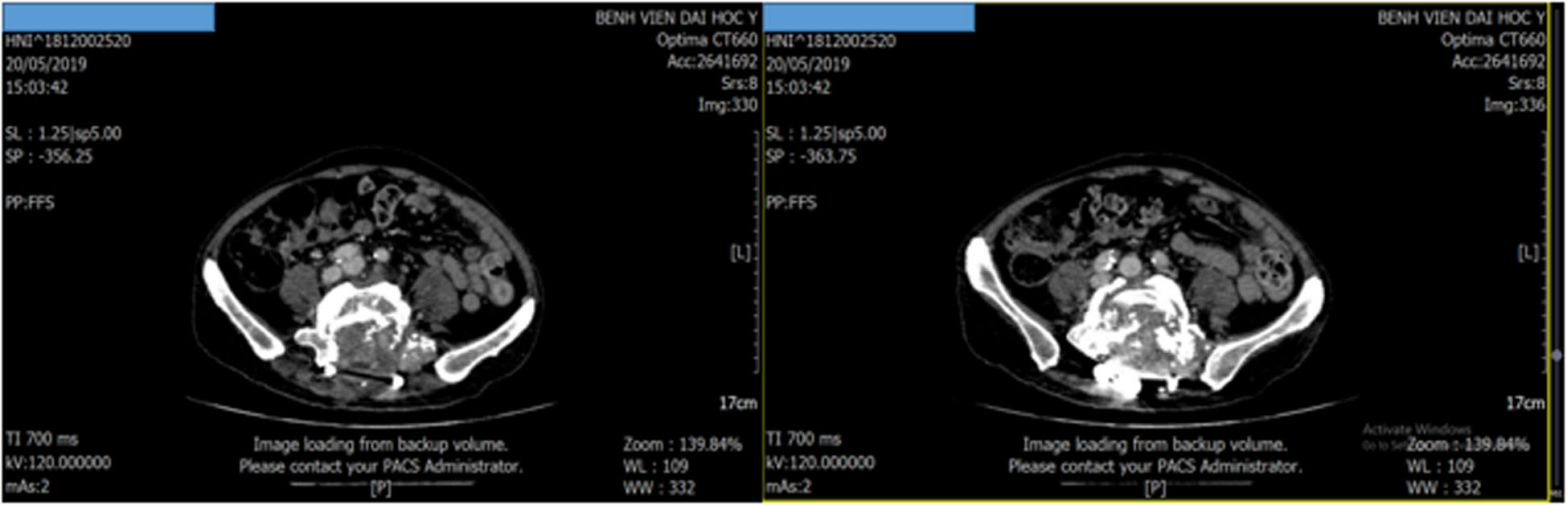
Abdominal computed tomography of patient at fifth month (May 2019)
